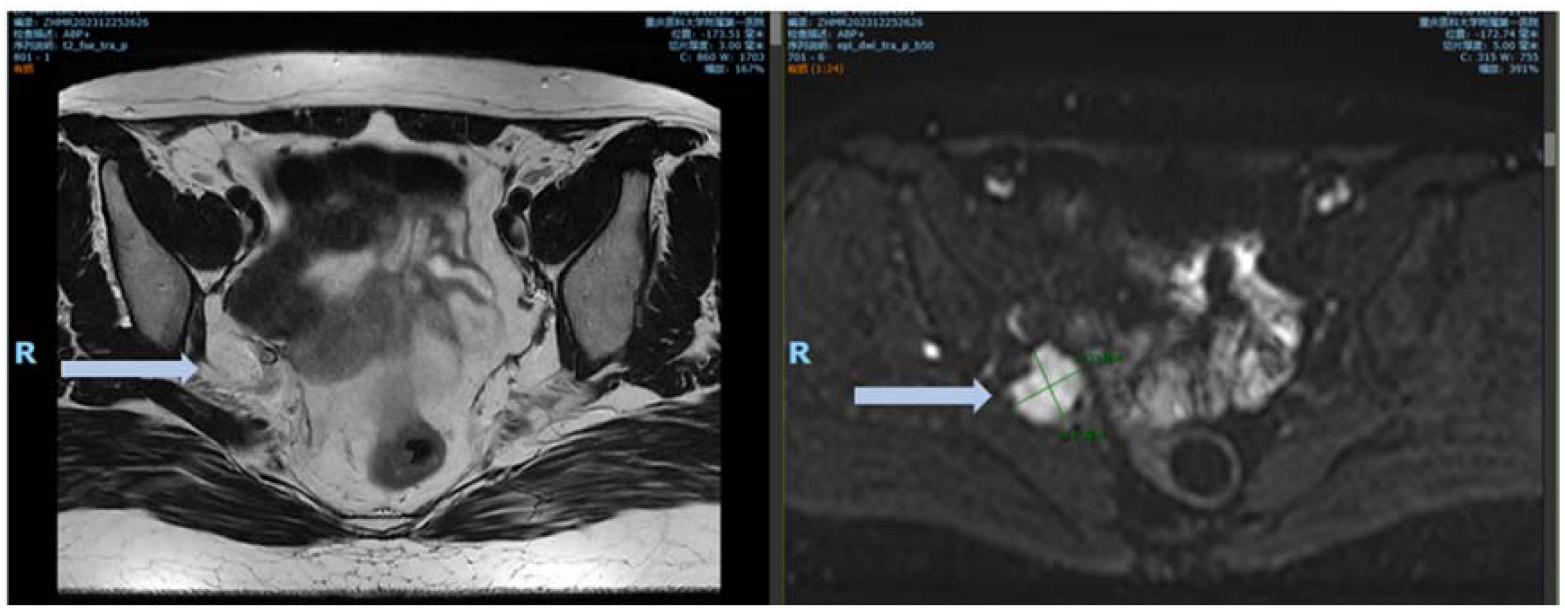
MRI images 8 months after surgery. DWI demonstrated an obvious high signal, with marked enhancement observed on the contrast-enhanced images.
